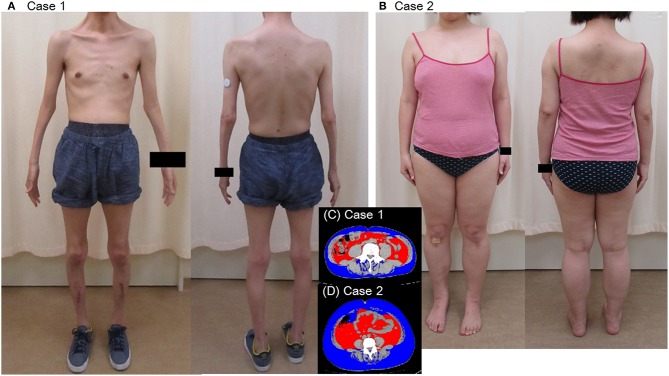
Appearance (A,B) and visceral fat area on CT of the patients (C,D).
